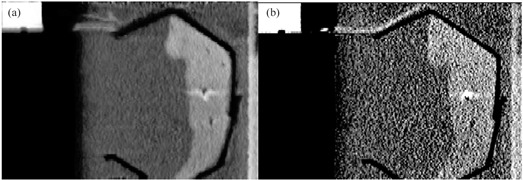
4D TDR reconstructed image (a) and according raw 4D CT image (b).
